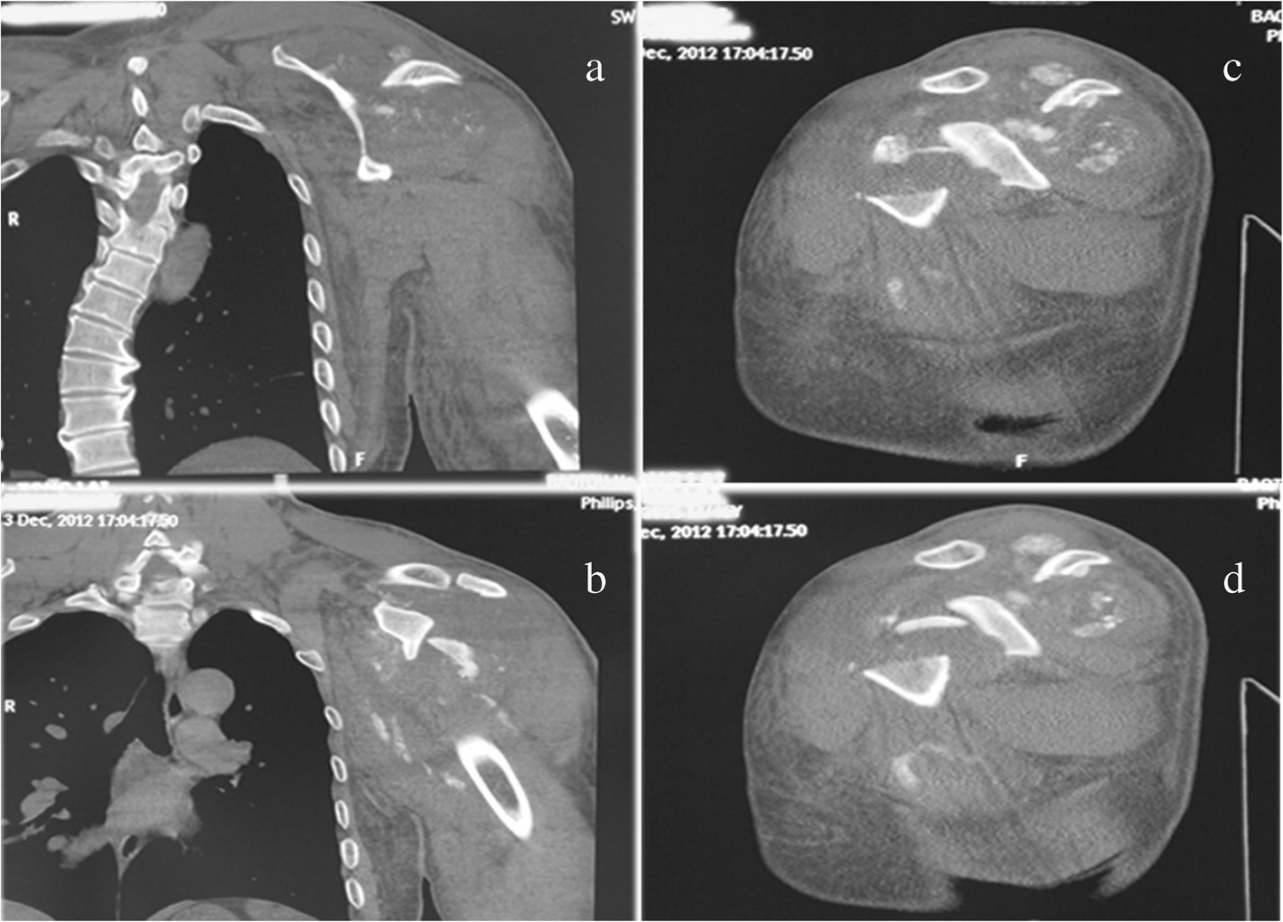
CT of this case. The right lateral curvature of the lower thoracic vertebra (a), broken humeral head and sporadic calcification shadow of the surrounding of this joint (b, c and d).
